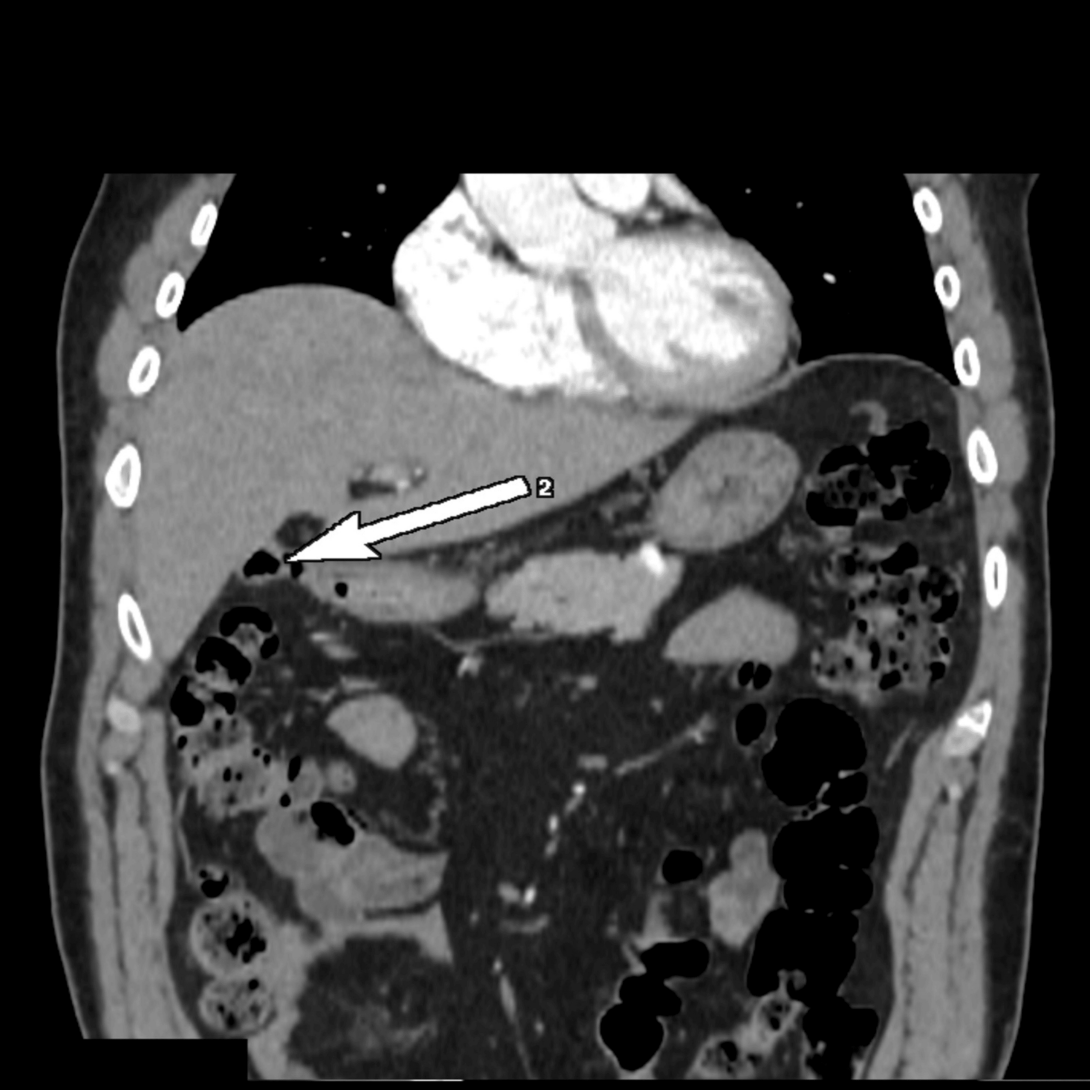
Computed tomography, coronary view; a fistula between the corpus of the gallbladder and the bulb of the duodenum (white arrow).
